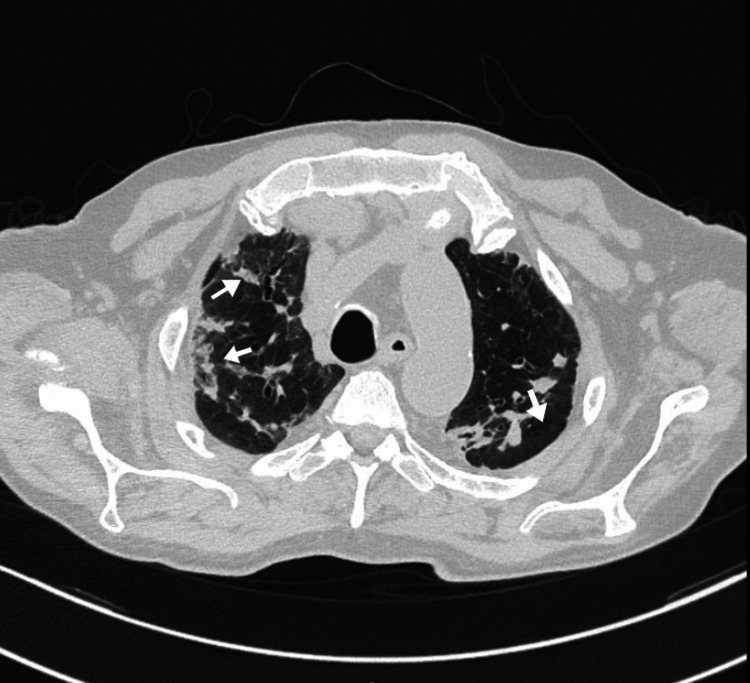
High-resolution computed tomography (HRCT) of the chest findings High-resolution computed tomography (HRCT) of the chest showing ground-glass opacity predominantly distributed in the peribronchovascular and subpleural regions.
